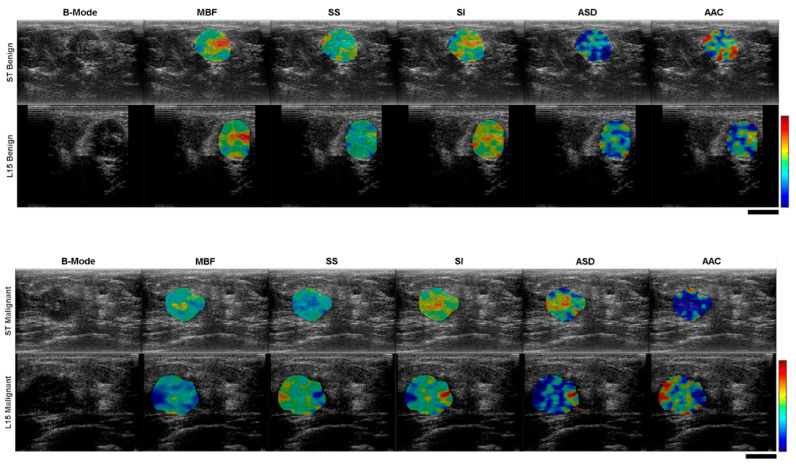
Cross-system comparison of ultrasound B-mode images with overlaid QUS feature parametric maps for representative benign (top) and malignant (bottom) clinical cases. The colour scale shows variation in feature magnitude. The black scale bars represent 1 cm.
